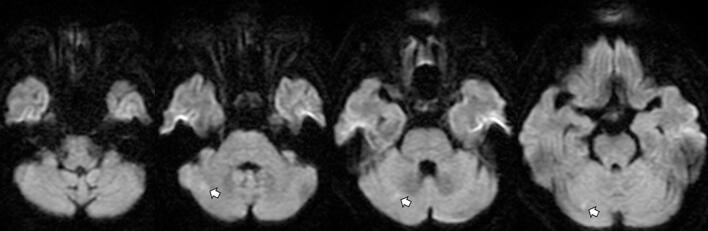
Diffusion-weighted magnetic resonance imaging showing acute cerebellar infarction. Diffusion-weighted MRI showing high signal intensity in the cerebellum, consistent with an acute infarction (arrow).
